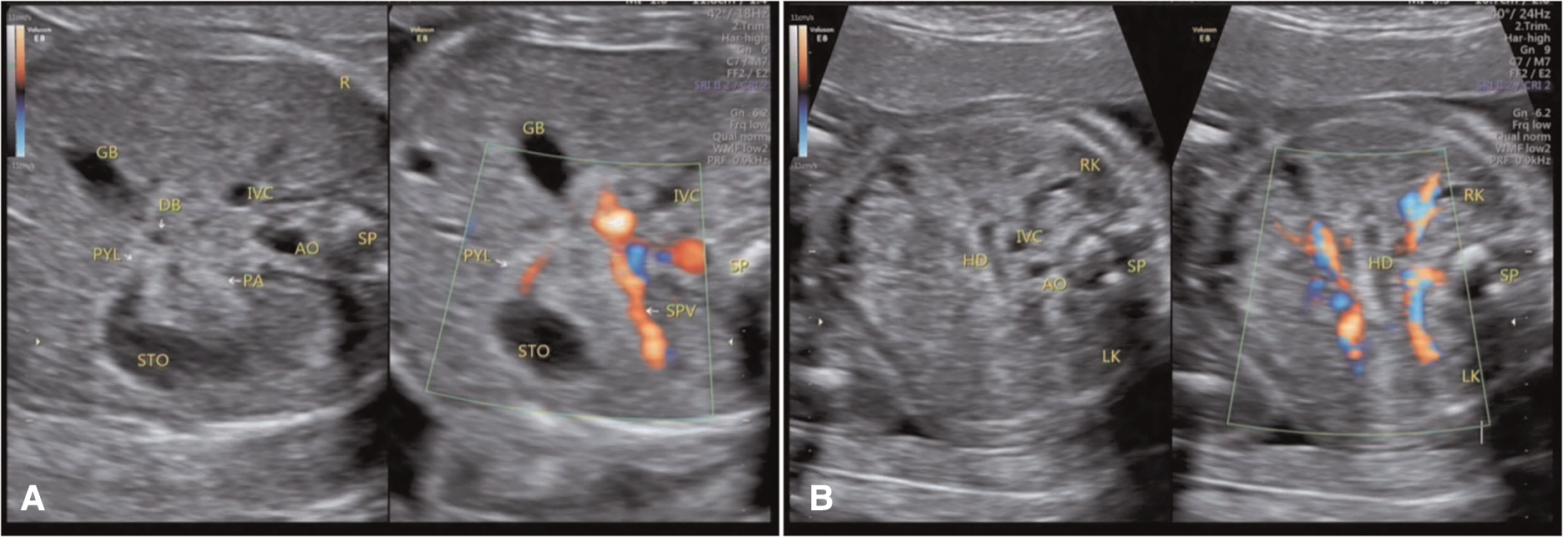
Color Doppler flow imaging of duodenum at 22 gestational weeks. (A) The moment the gastric contents passed through the pylorus. (B) The filling state of the horizontal part of the duodenum. STO, stomach; PYL, pylorus; DB, duodenal bulb; HD, horizontal part of the duodenum; AO, abdominal aorta; IVC, inferior vena cava; SPV, spleen vena; GB, gall bladder; PA, pancreas, RK, right kidney; LK, left kidney; SP, spine; R, right side of the fetus.
